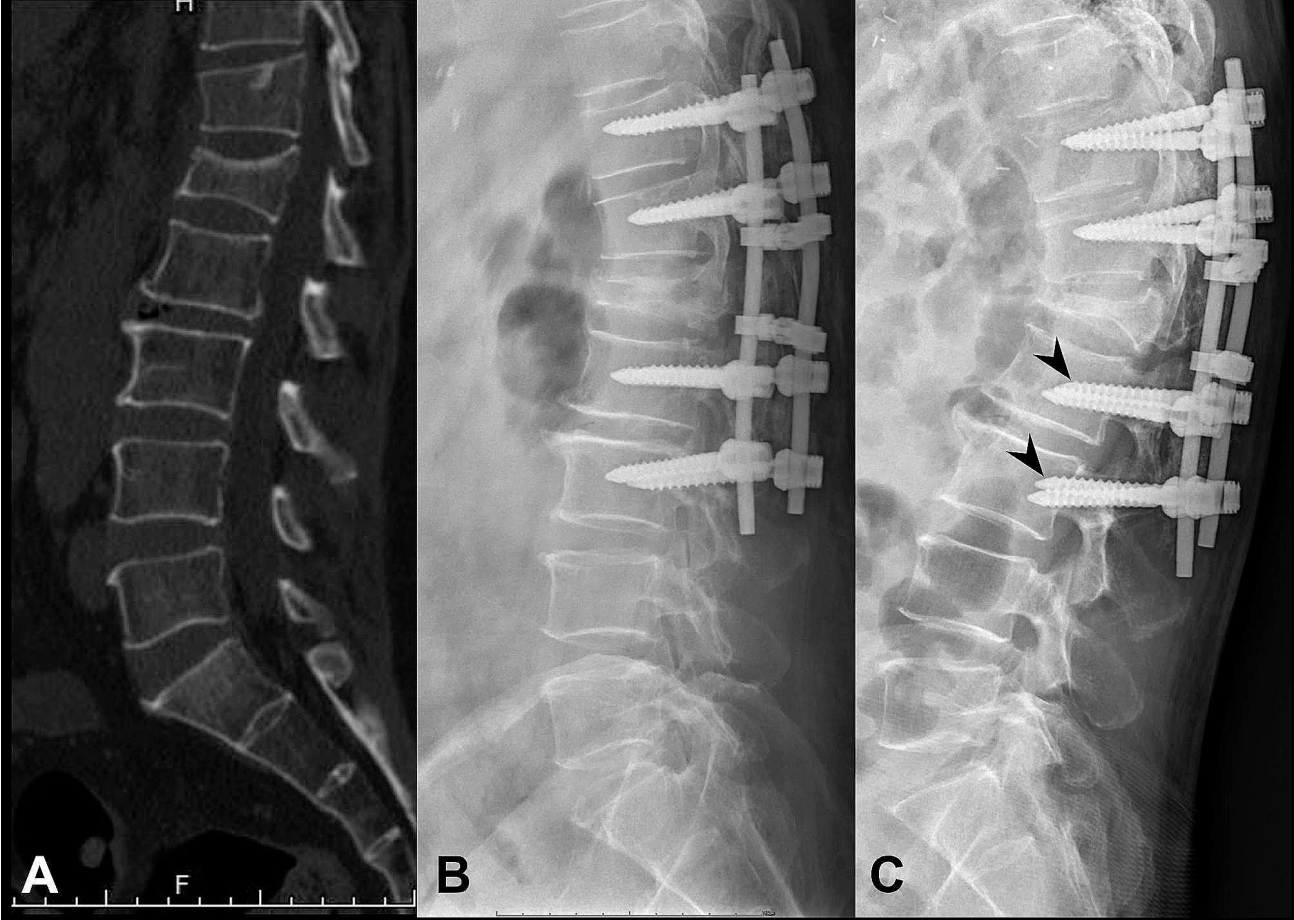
A 62-year-old male patient with an A3-type burst fracture at the L1 level and a McCormack load-sharing score of 5 underwent long-segment pedicle screw fixation. A: Preoperative lateral spine computed tomography scan. B: Postoperative lateral spine radiography on day 1. C: Inferior pedicle screw pullout (arrowhead) seen in lateral spine radiography at the 1-month postoperative follow-up
